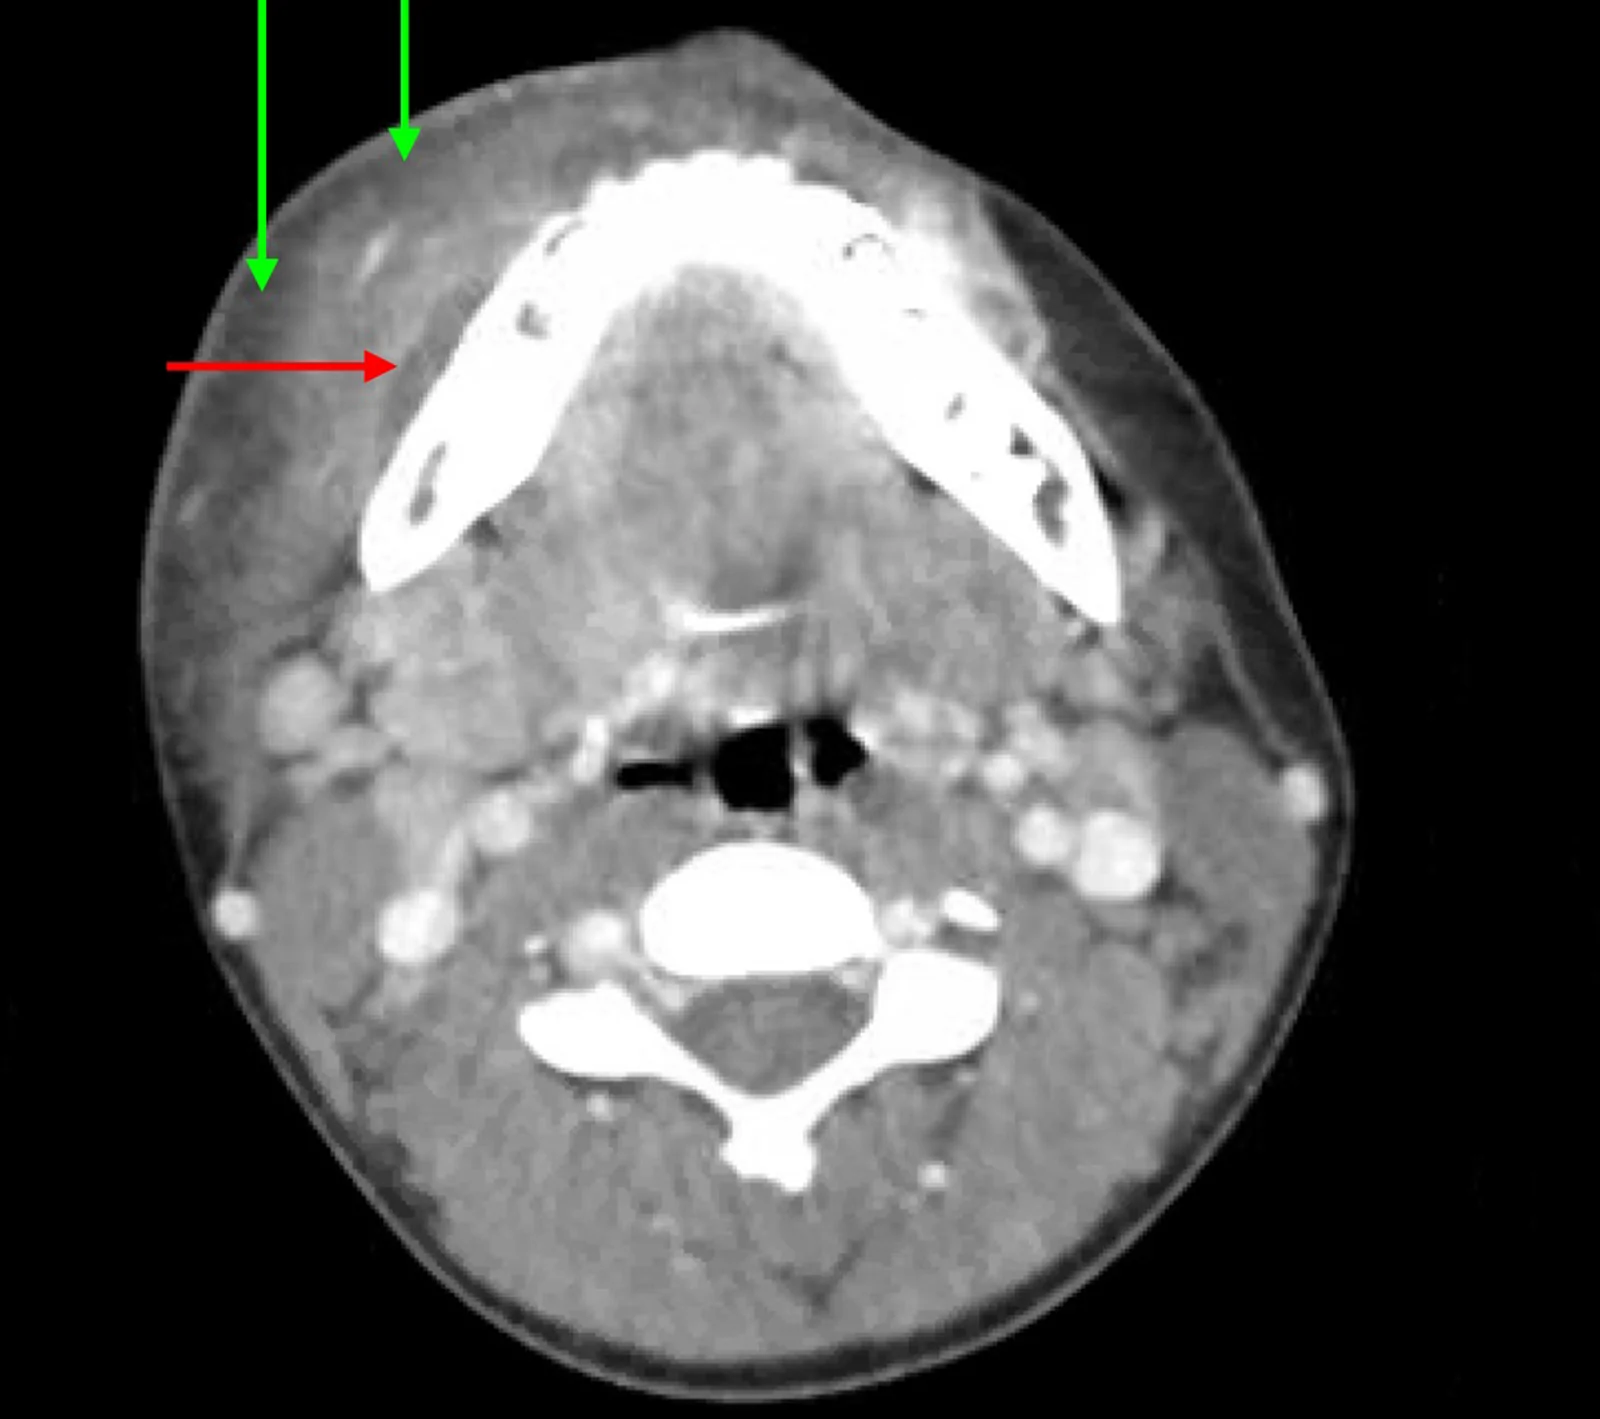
Axial view of CT scan—soft tissue of the neck. The red arrow denotes the subperiosteal abscess and the green arrow denotes the facial cellulitis. CT, computed tomography.
